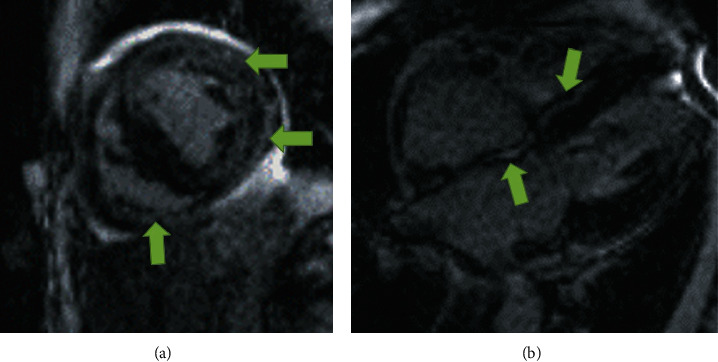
Delayed gadolinium enhancement cardiac MRI images. The image on (a) shows a short axis view, (b) is a 4-chamber view. Extensive midmyocardial and atrial wall enhancement secondary to amyloid deposition is noted (green arrows) which is a signature of cardiac amyloidosis.
